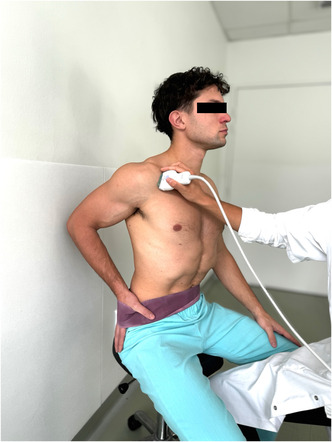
Based position for dynamic exploration with Pilates elastic band. For dynamic exploration under controlled resistance, the patient is positioned identically to the standard method. A Pilates elastic band (purple arrow) is firmly secured around the trunk to provide uniform counter‐resistance during movement. The patient slides their hand under the band with their thumb placed externally (red star), which maintains proper alignment and prevents compensatory movement of the wrist or elbow. Ultrasound probe is oriented in the coronal oblique plane, in the deltopectoral groove (dashed black arrow).
